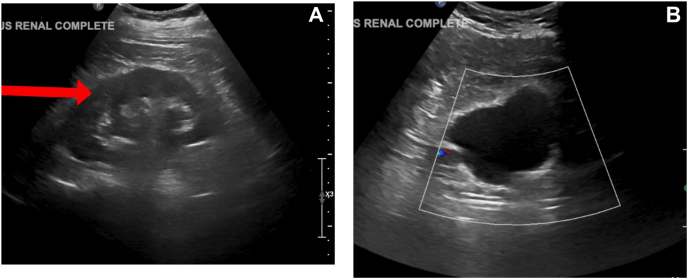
(A) Renal ultrasound long-axis view at initial presentation demonstrating mild to moderate hydronephrosis of the right kidney. (B) Bladder ultrasound transverse view at initial presentation demonstrating normal bladder morphology without visible obstruction.
